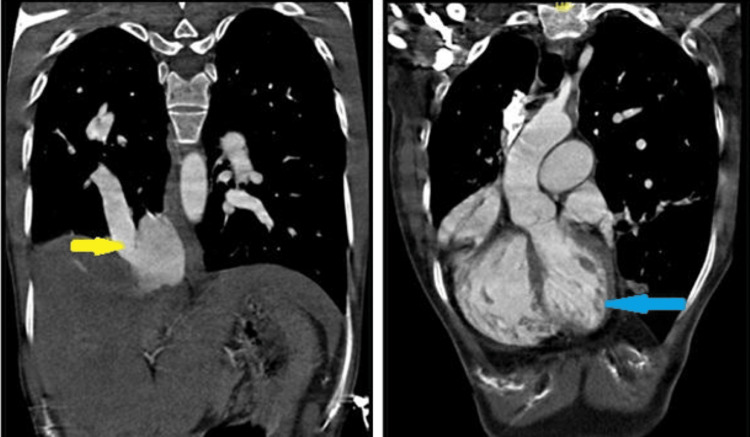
Coronal view of CT scan Left panel: Scimitar vein opening at IVC-RA junction (yellow arrow); Right panel: Dextro-posed heart shifted into right hemithorax (blue arrow) IVC: Inferior vena cava, RA: Right atrium
